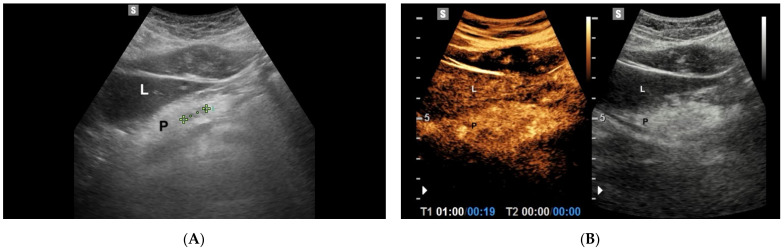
Focal autoimmune pancreatitis. (A) B mode Ultrasonography shows a 1.2 cm hypoechoic lesion (calipers) in the pancreatic body; (L: liver; P: Pancreas). (B) On CEUS, in the arterial phase (19 s after the injection of c.a.), the lesion is isoechoic in comparison with the adjacent pancreatic parenchyma.
